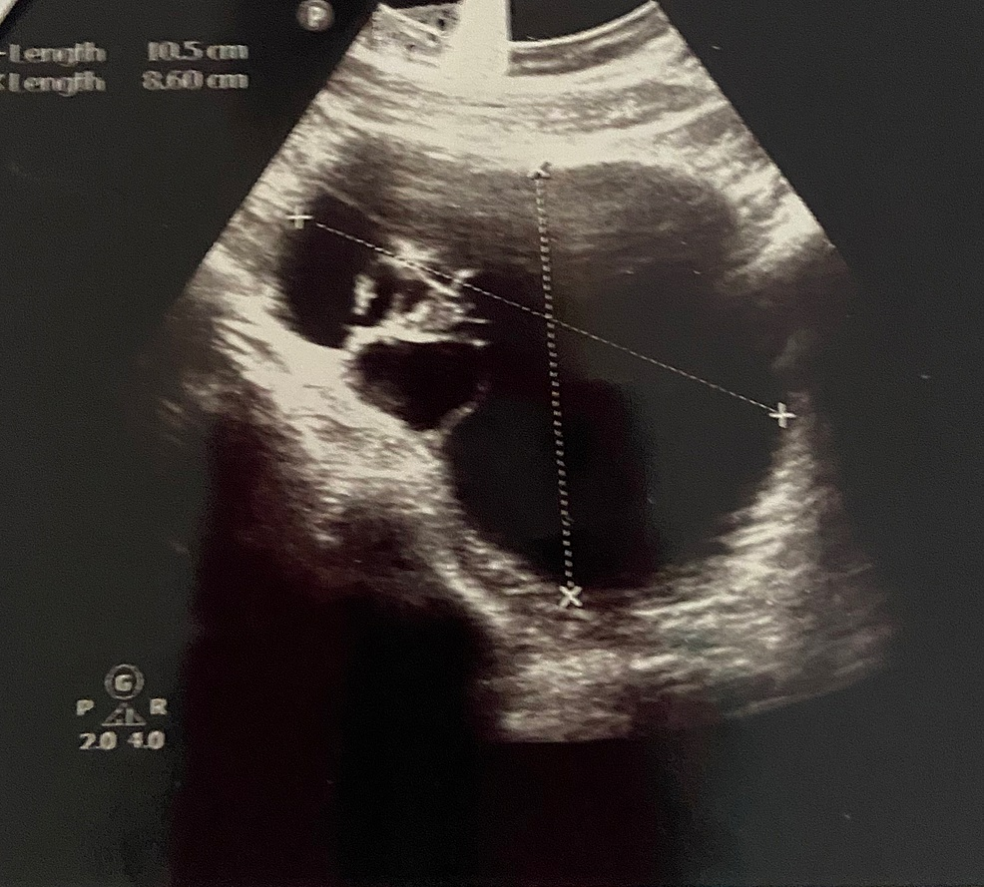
Transvaginal ultrasound revealing the right adnexal cyst
